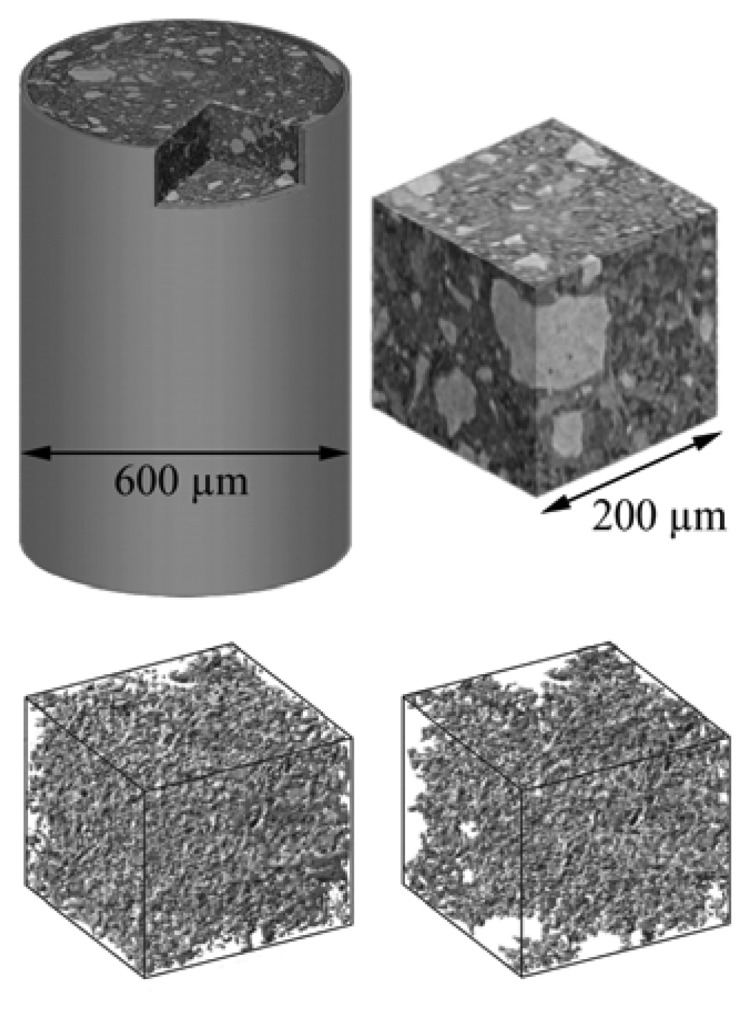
Refractive index microradiology implemented in the tomographic mode: computer-reconstructed images derived from a large number of projection radiographs. The top images (data from Ref. 27) were obtained for a 1 day old cement sample and show a reconstructed rendering of the entire sample as well as that of a specific volume. The bottom images – referring to a 3 day old sample - show how computer processing can yield reconstructed images of the pores (bottom left) and of the fraction of the pores that are interconnected or “percolating”.
